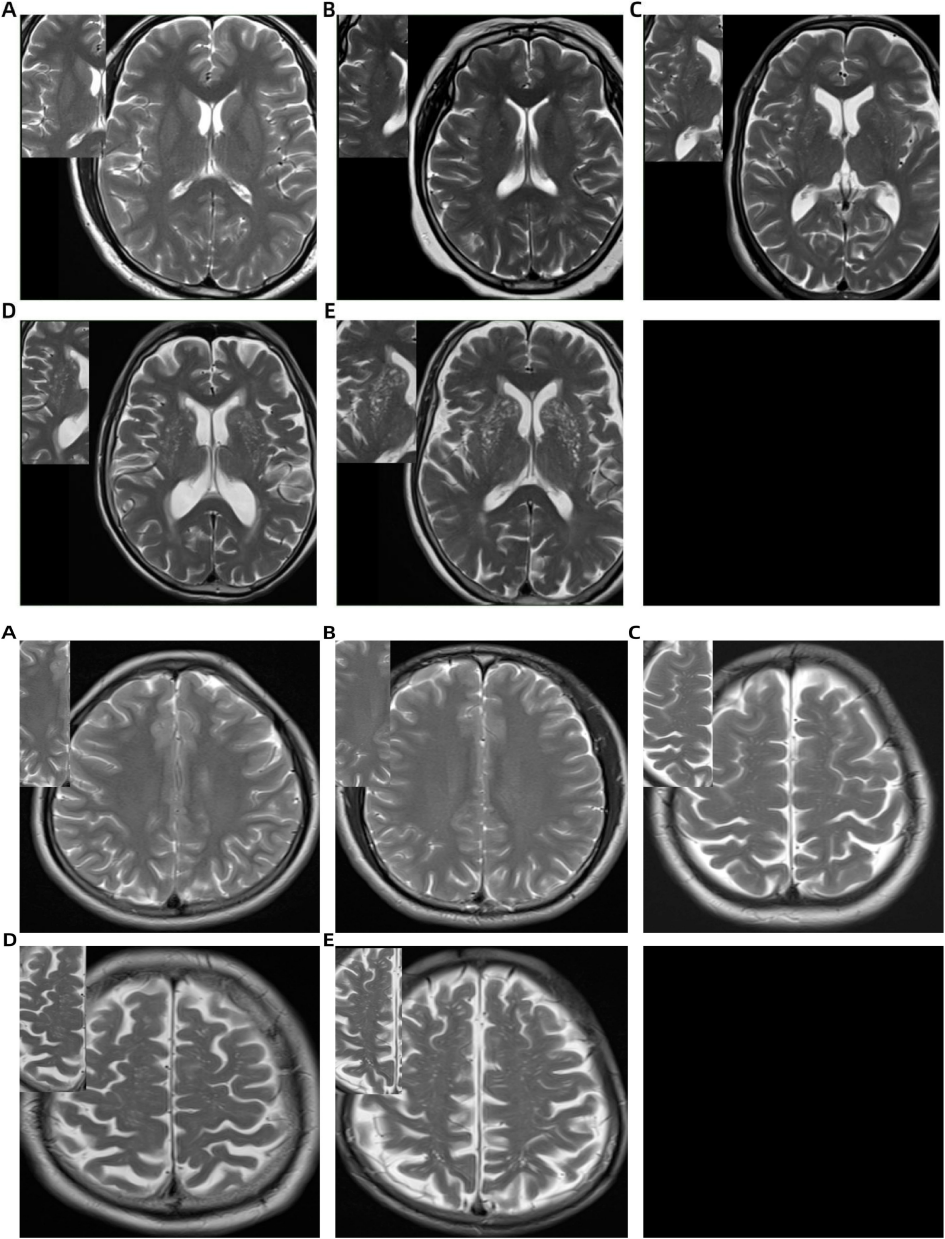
Basal ganglia (BG) and centrum semiovale (CSO) enlarged perivascular space (EPVS) scoring system on T2-weighted MRI: (A) no EPVS (score = 0); (B) 1–10 EPVS (score = 1); (C) 11–20 EPVS (score = 2); (D) 21–40 EPVS (score = 3); (E) >40 EPVS (score = 4).
